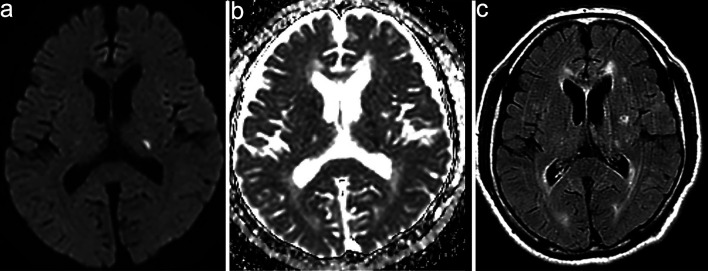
Magnetic resonance imaging (MRI) on admission. (a) High-intensity lesion in the left thalamus on diffusion-weighted imaging (DWI). (b) Corresponding low-intensity lesion on the apparent diffusion coefficient (ADC) map. (c) Fluid-attenuated inversion recovery (FLAIR) images demonstrating multiple spotty hyperintense white matter lesions.
